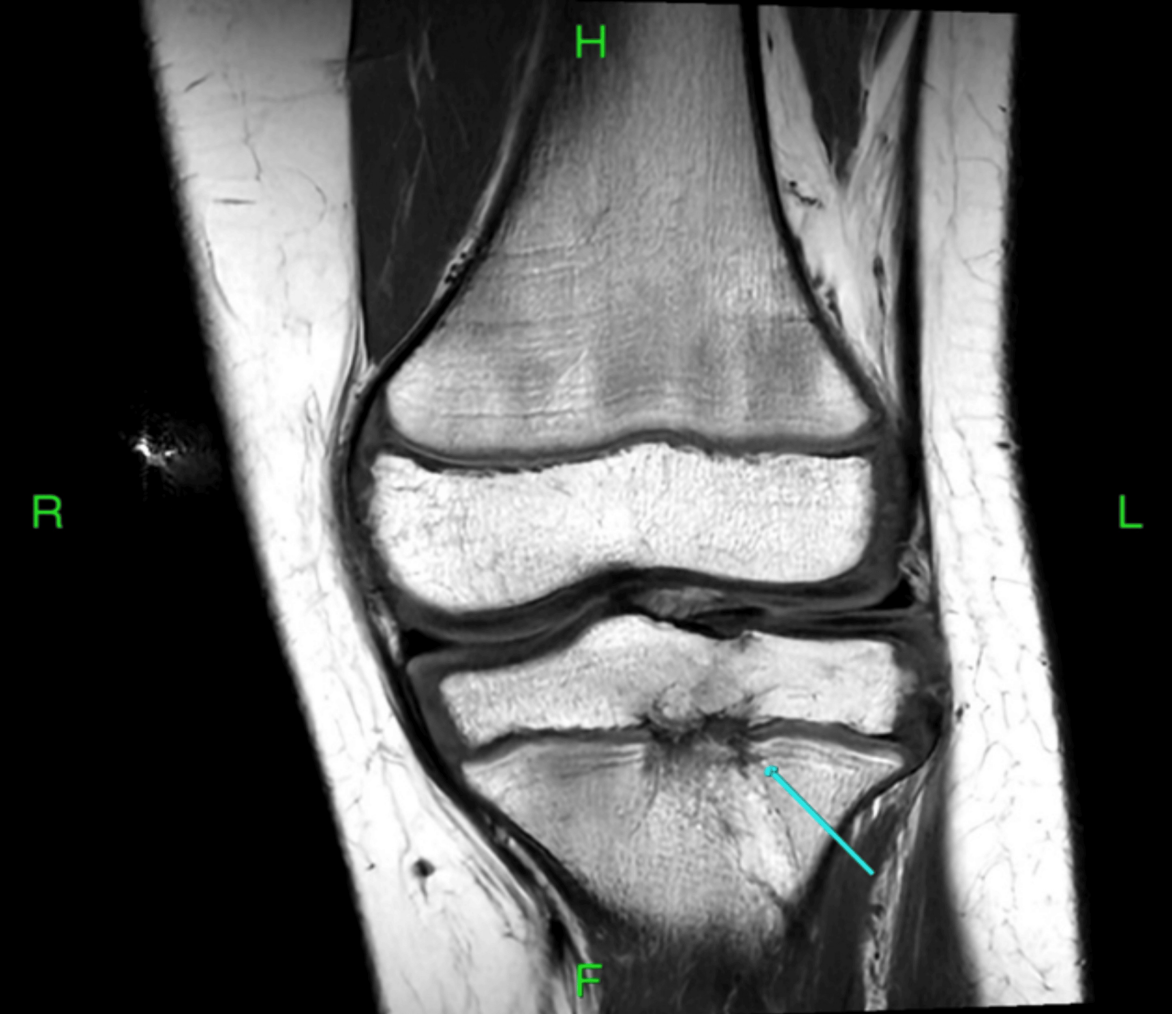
Coronal T2-weighted MRI image showing the absence of the lesion, verifying complete resection of the abscess MRI: magnetic resonance imaging
